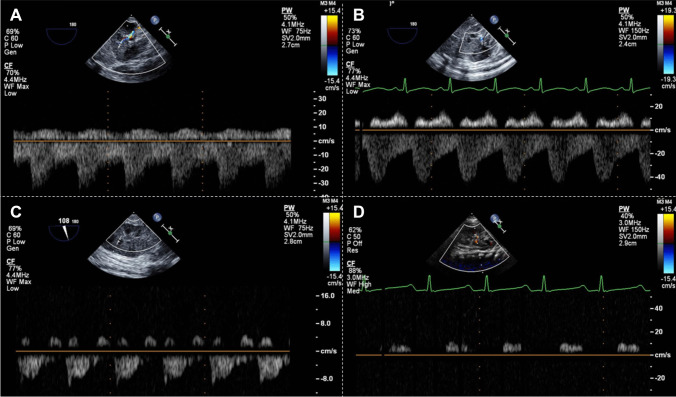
Four types of intrarenal venous flow Doppler waveform assessed via transesophageal echocardiography. A, continuous flow; B, pulsatile flow; C, biphasic flow; D, monophasic flow. Patterns A and B were classified as pattern 1, C as pattern 2, and D as pattern 3
